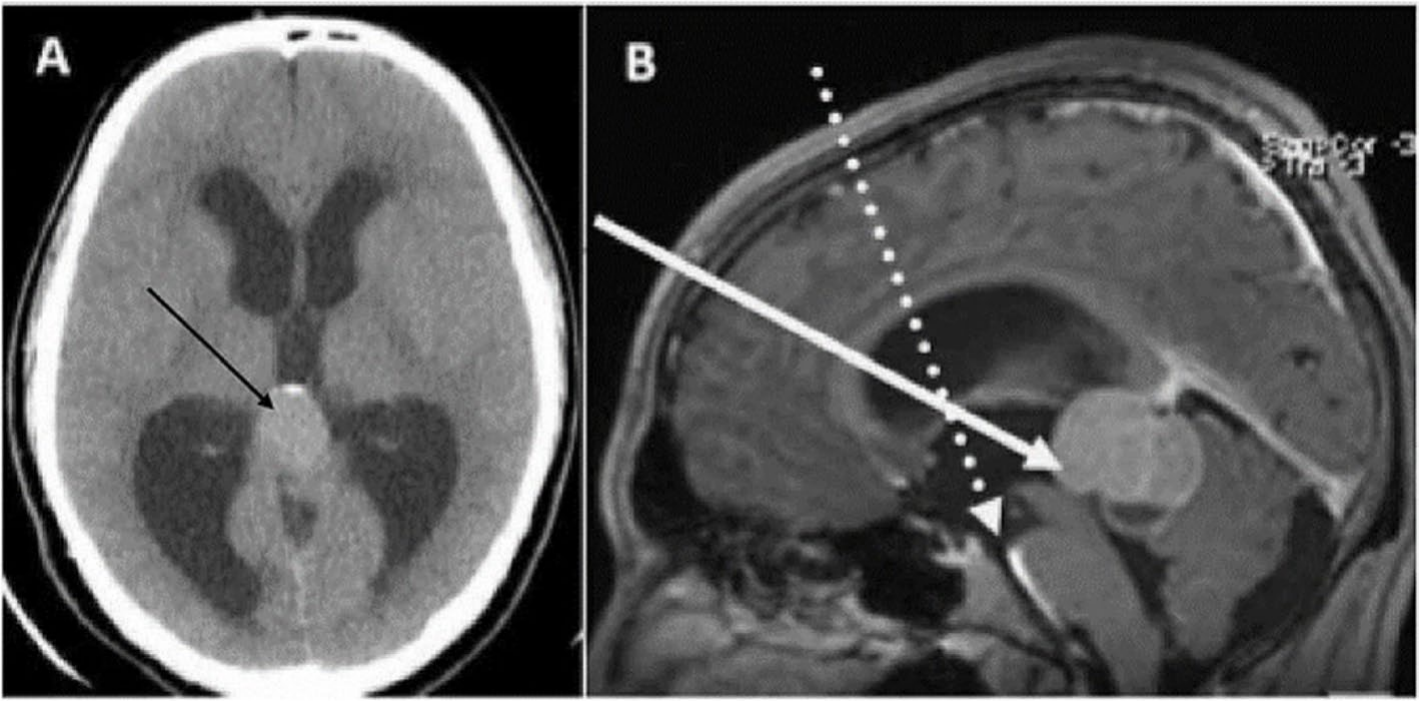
A Preoperative plain CT brain showing the lesion (black arrow). B Preoperative sagittal T1 MRI with contrast showing the planned endoscopic trajectories. The first trajectory was for third ventriculostomy (dashed arrow) (via the right lateral ventricle and the right foramen of Monro towards the target point in the midline of the floor of the third ventricle, 3 mm in front of the mamillary bodies), and the second trajectory (solid arrow) was for tumor biopsy (via the right frontal horn and the right foramen of Monro towards the midpoint of the tumor)
